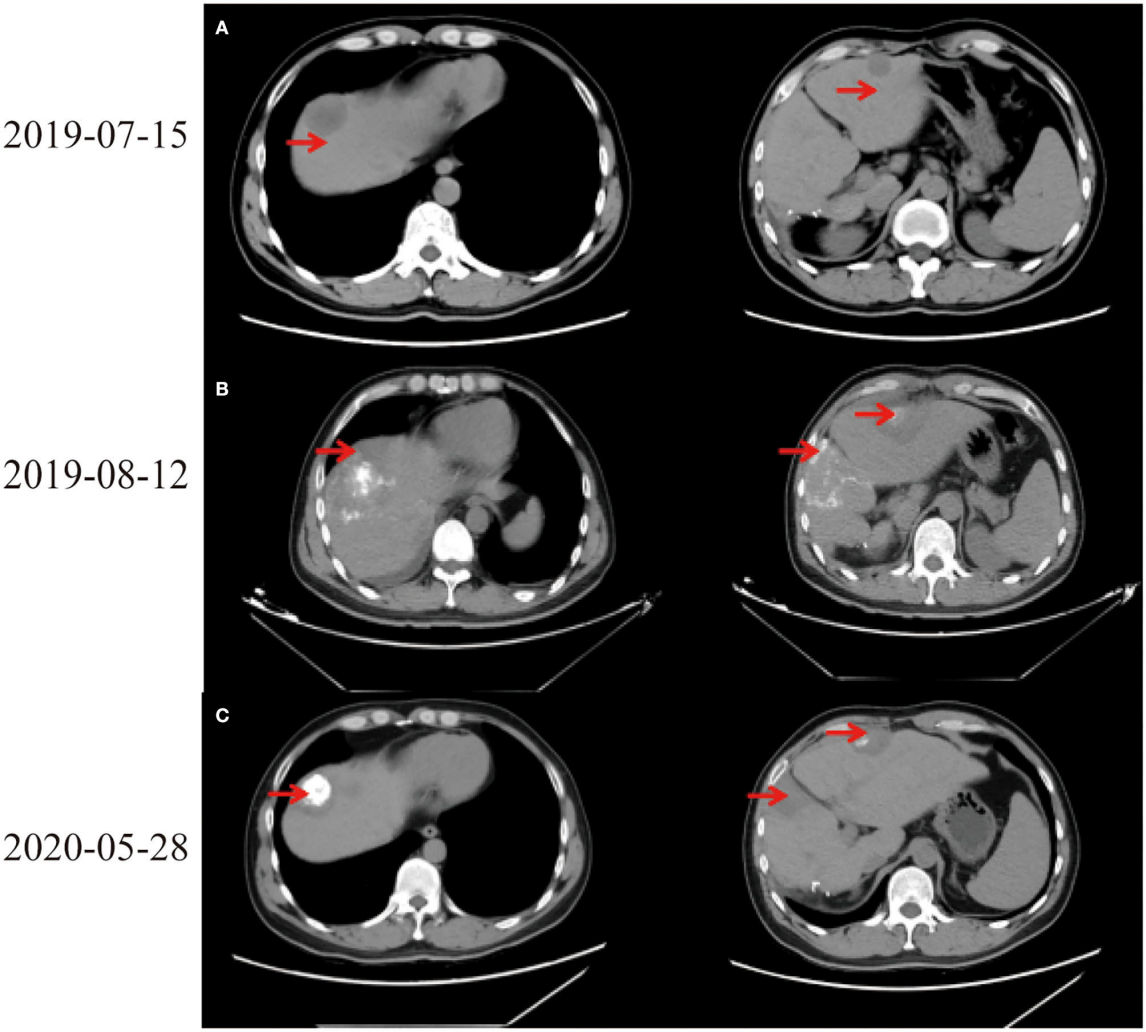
(A–C) The imagines of chest and abdomen by CT.
